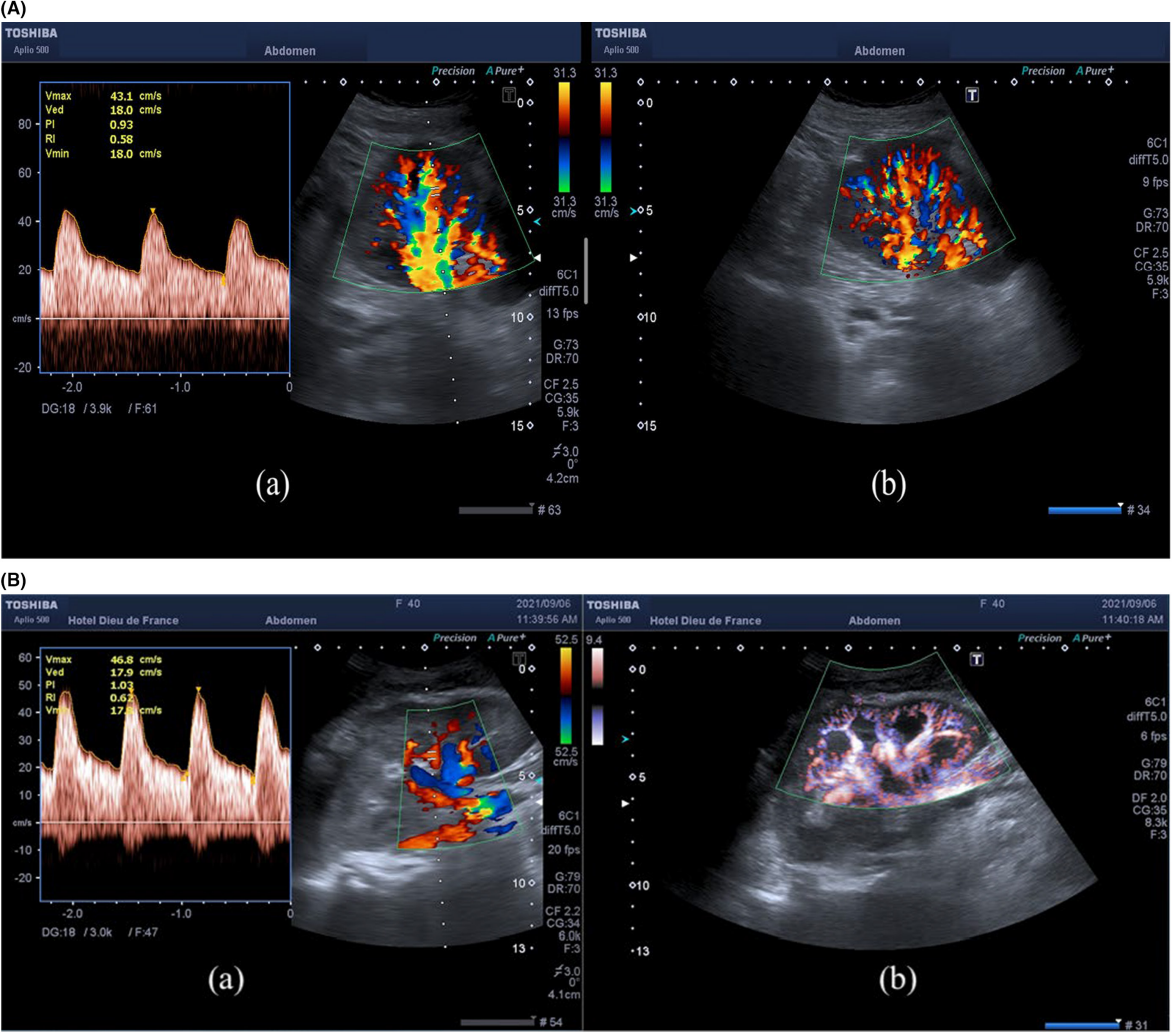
(A) Patient A: (a) spectral waveform of intrarenal arteries shows a complete return to normal of the resistive index, evaluated at 0.58. (b) Sagittal view of the graft shows a complete resolution of the mass effect and normal Color Doppler vascularization of the renal parenchyma. (B) Patient B: (a) spectral waveform of intrarenal arteries shows a complete return to normal of the resistive index, evaluated at 0.62. (b) Sagittal view of the graft shows a complete resolution of the mass effect and normal Color Energy vascularization of the renal parenchyma.
